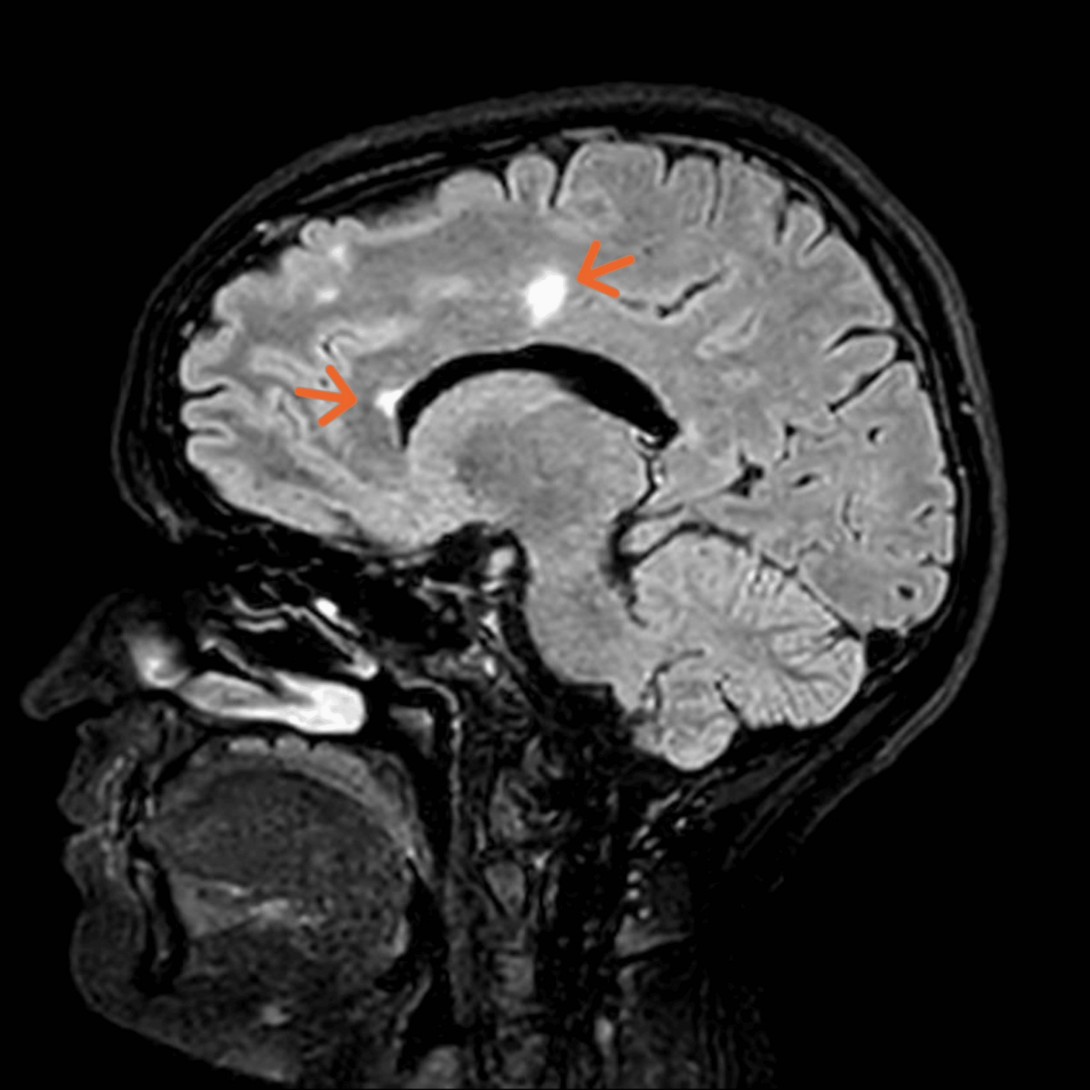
Sagittal midline MRI FLAIR image of the head showing oval-shaped periventricular and peri-callosal variable sized lesions. MRI: magnetic resonance imaging FLAIR: fluid-attenuated inversion recovery
